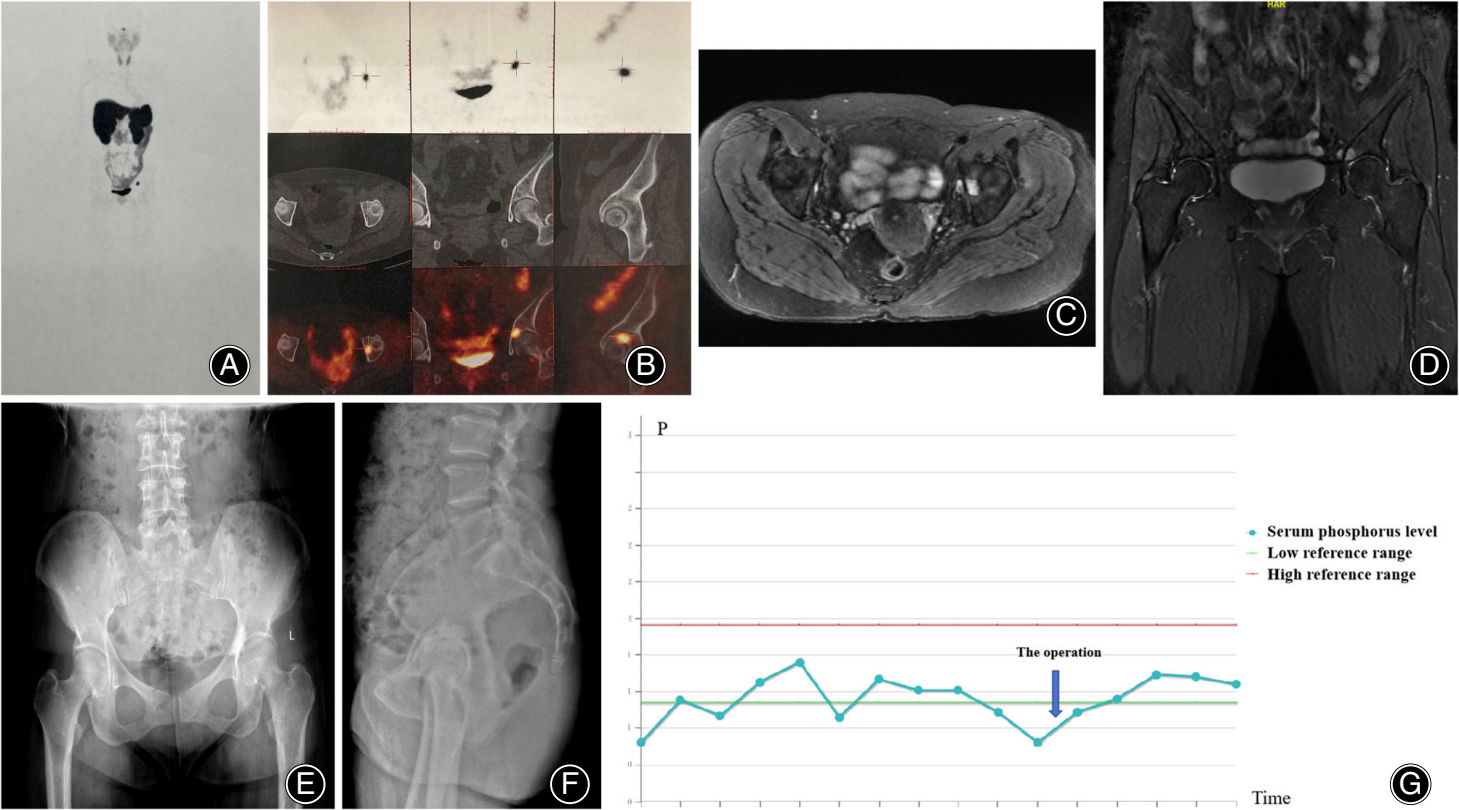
Case 1 (A) The octreoscan showing the suspected culprit tumor in left acetabular region. (B) The ^68^Ga‐DOTATATE PET/CT revealing high intake in the left acetabulum. (C, D) MRI of the hip revealing the mass, which was highly indicative of the causative tumor. (E, F) The postoperative X‐ray of the pelvis. (G) Serum phosphorus levels elevated to the normal range after the operation.
